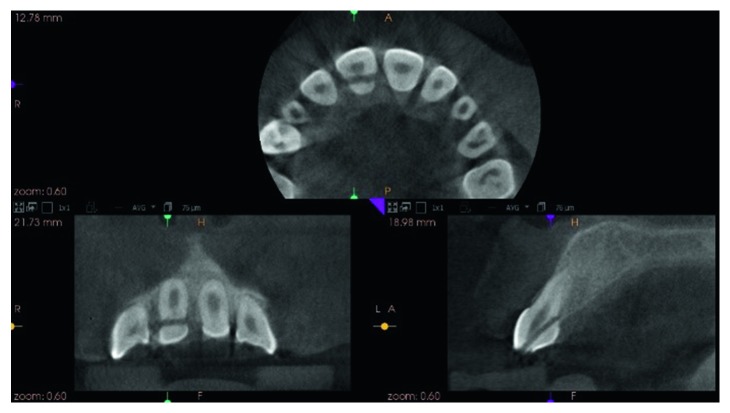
Cone beam computed tomographic view of tooth 11 in various planes showing the extent of the fracture.
